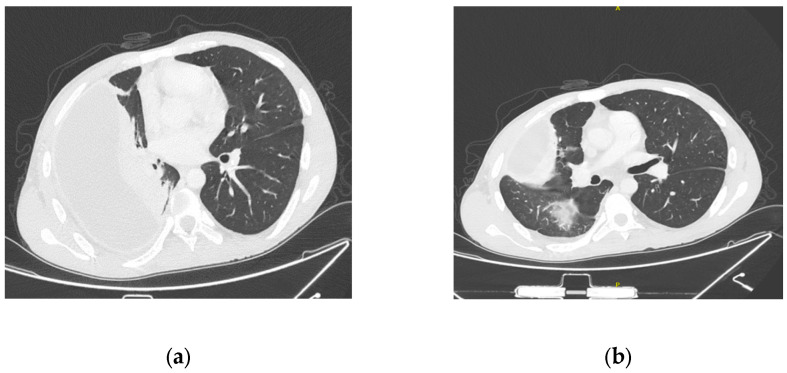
The admission chest CT scan shows a massive right pleural empyema (a) with pulmonary infiltrates (b).
